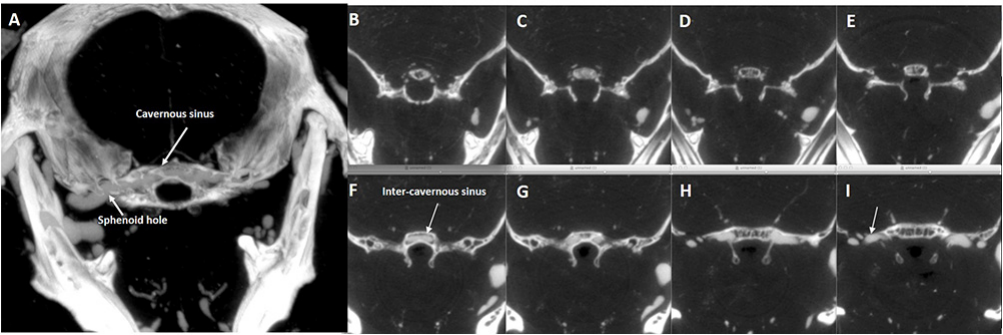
Axial computed tomography of the middle cranial fossa. Axial computed tomography slices at the level of the middle cranial fossa of the basicranium; (A) = 1mm-thick “MIP-ped” slab; (B)-(I) consecutive 0.05mm-thick slices in a cranio-caudal sequence showing the confluence of small vessels in the hypothalamic region (B), (C), (D) toward a large intraosseus median inter-cavernous sinus (E), (F) which bilaterally exits the brain (G), (H) to join the external jugular vein system (arrow in (I)).
